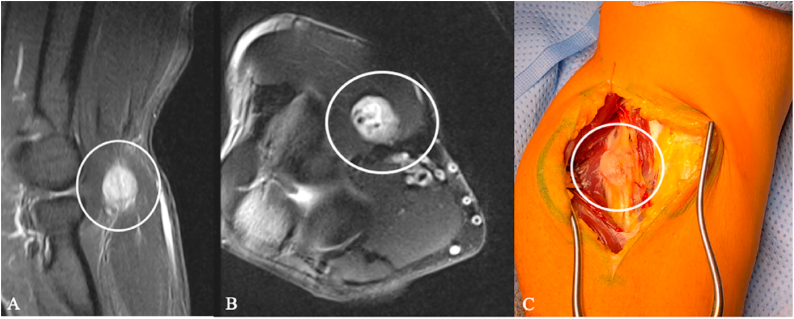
Fibroblastic soft-tissue tumor of the radial nerve. (A, B) MRI and intraoperative findings in a 66-year-old man showed a circumscribed but inhomogeneous mass (circle) along the right radial nerve at the elbow, initially suspected to be a neurofibroma (C) intraoperatively the lesion was infiltrative and fascicle-engulfing, requiring complete resection with sural-nerve interposition graft reconstruction, and the patient had marked preoperative finger extensor weakness with motor and sensory deficits present both before and after surgery.
